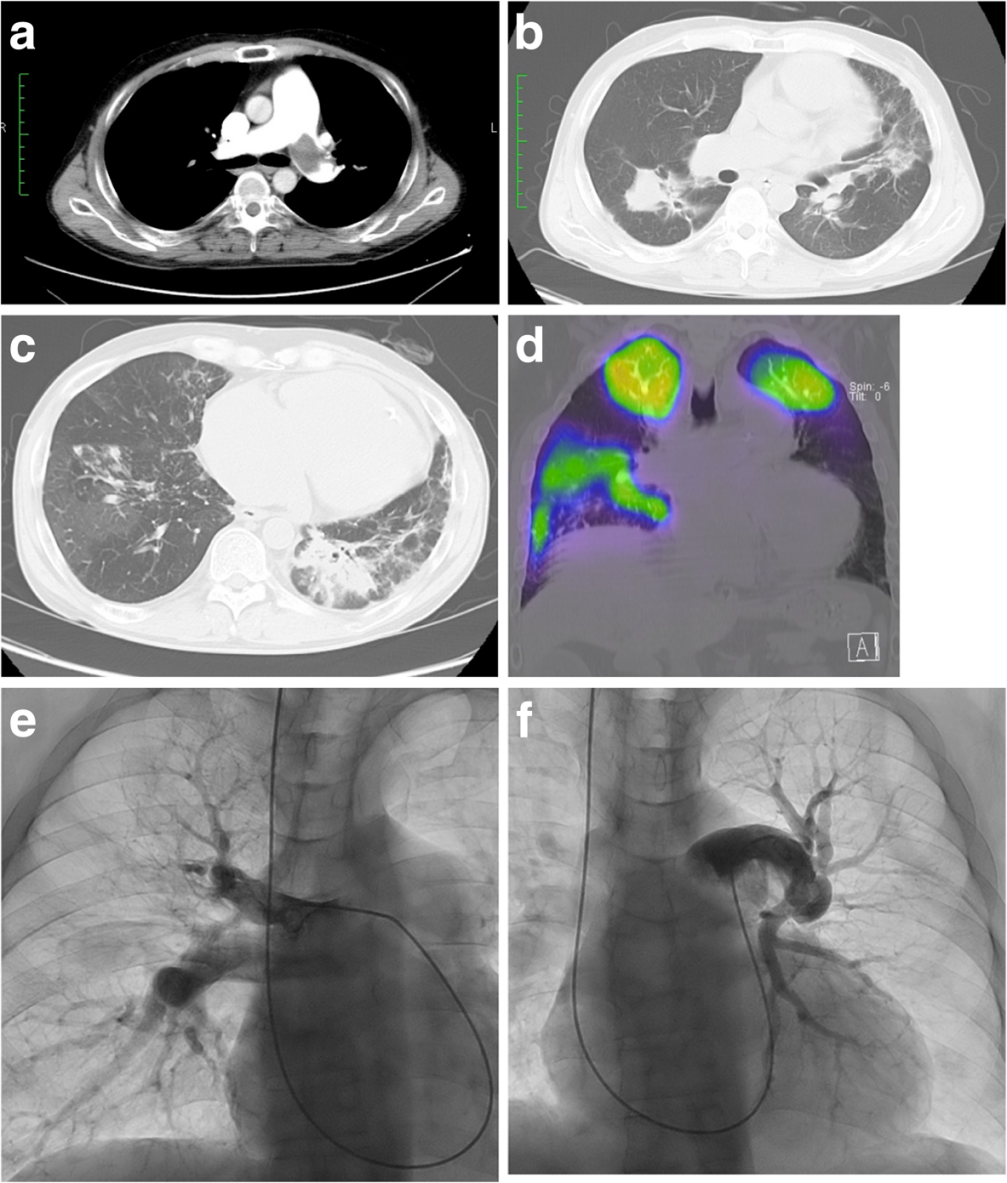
a Preoperative computed tomography scan of the lung showing showed proximal chronic pulmonary emboli. b Preoperative computed tomography scan of the lung showing a nontuberculous mycobacterial infection. c Preoperative computed tomography scan of the lung showing aspiration pneumonia. d Preoperative lung perfusion scintigraphy showing multiple segmental defects. e, f Preoperative pulmonary artery angiograms showing intimal irregularities and abrupt narrowing of both pulmonary arteries
